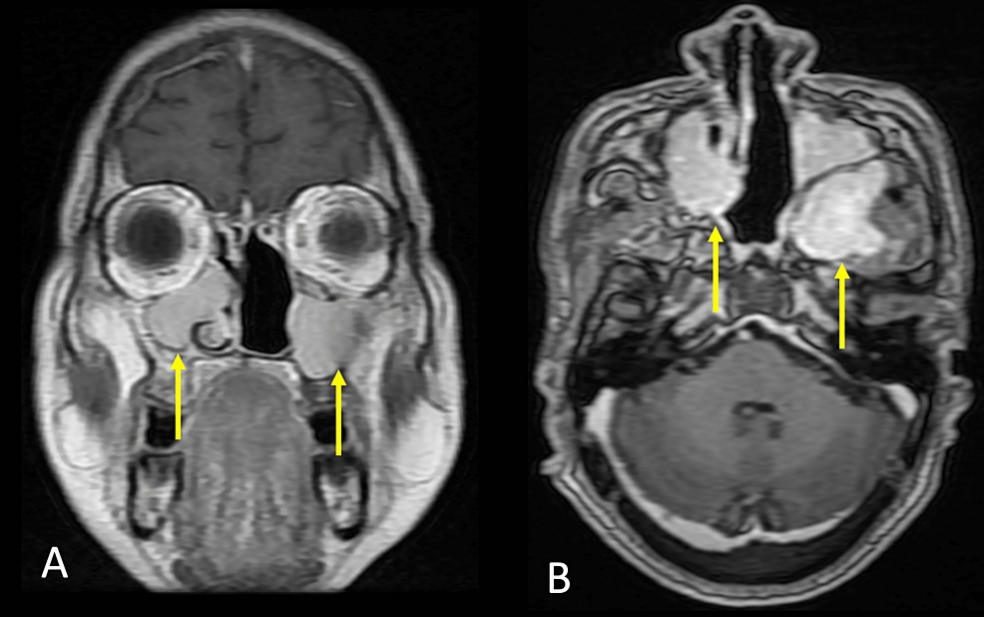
Magnetic resonance imaging with contrast A. Coronal contrast section showing sinonasal involvement (yellow arrow). B. Axial contrast section showing bilateral maxillary sinus, left sphenoid sinus involvement (yellow arrows).
